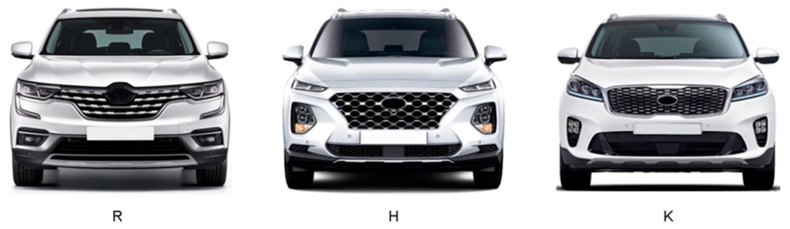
SUV images for the experiment.
